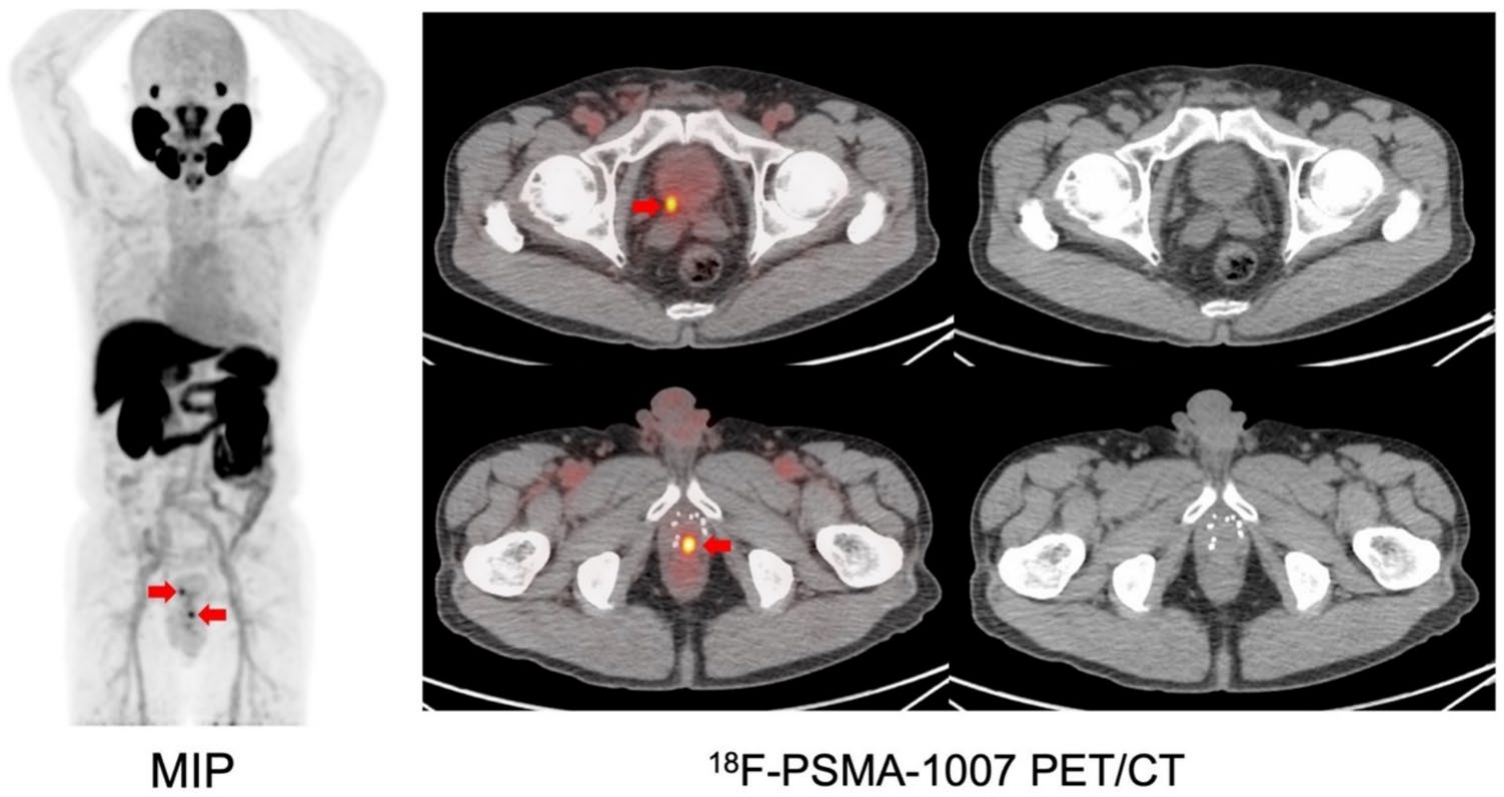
[^18^F]PSMA-1007 PET in patient with biochemical recurrence after ^125^I-seed implantation (PSA at PET: 3.32 ng/mL) (MIP: maximum intensity projection). Focal uptakes are observed on PSMA–PET (red arrows) and biopsy on the caudal lesion revealed a recurrence. Radiation therapy (cyber-knife) is performed targeting the two lesions and PSA value shows a decrease. (Cited from reference no. 46 in accordance with the open access policy.)
